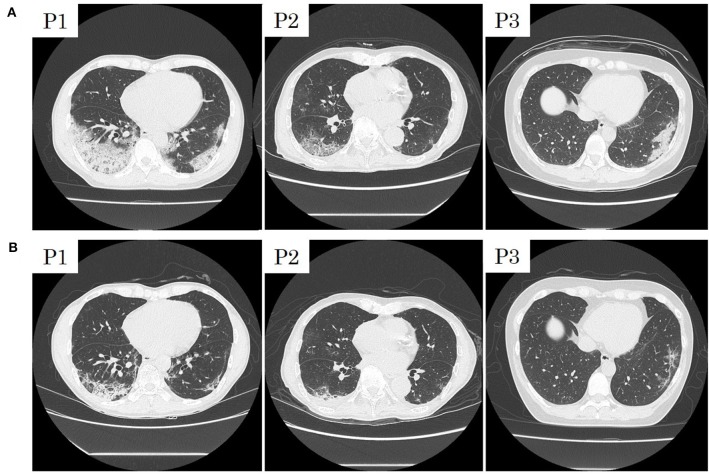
Initial computed tomographic images of patients 1 (P1), 2 (P2), and 3 (P3) with COVID-19 pneumonia (A) and those after treatment with Lopinavir/Ritonavir (B).
